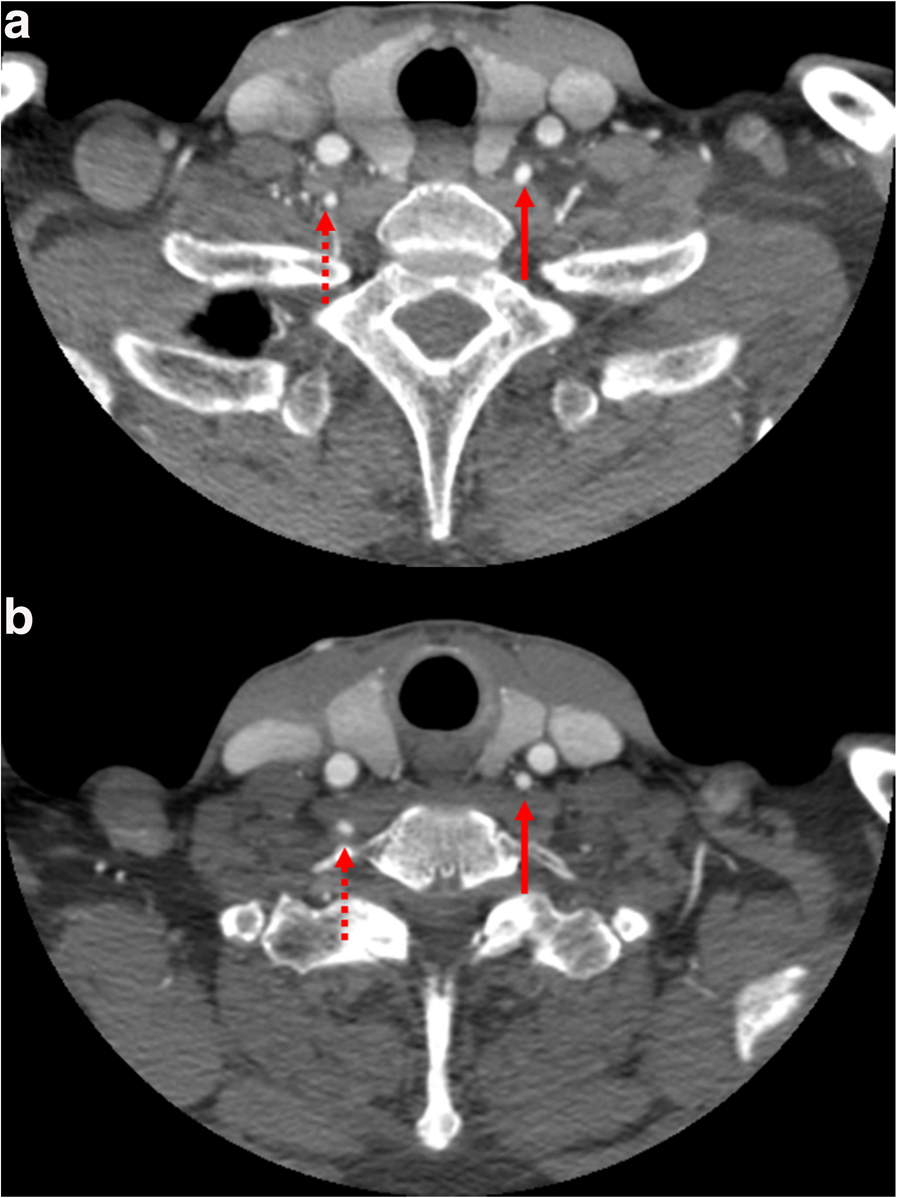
a, b Preoperative neck computed tomographic (CT) angiography. Solid arrow: the aberrant left vertebral artery; dotted arrow: the right vertebral artery
